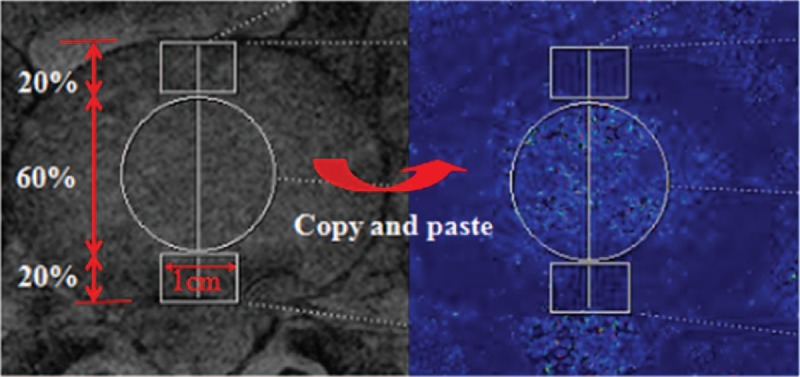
MRI T2WI (first echo image) a region of interest (ROIs) for IVDs were drawn on the axial. All ROIs were selected on the morphological images and transferred via “copy and paste” into the T2∗ maps.
